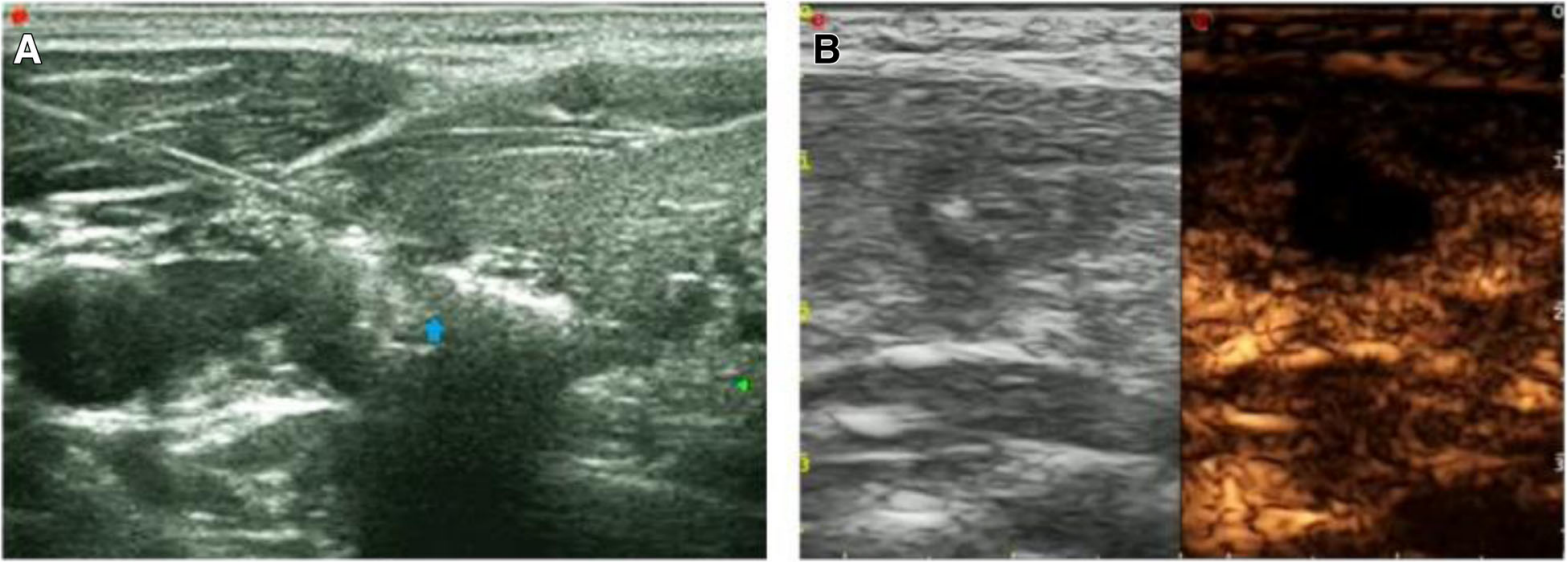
a During the course of PLA under ultrasound guidance, the lesion was carbonized with the release of ablation energy, and a hyperechoic area could be observed. b CEUS was conducted are the completion of PLA, and a hypoechoic area representing complete ablation could be observed
